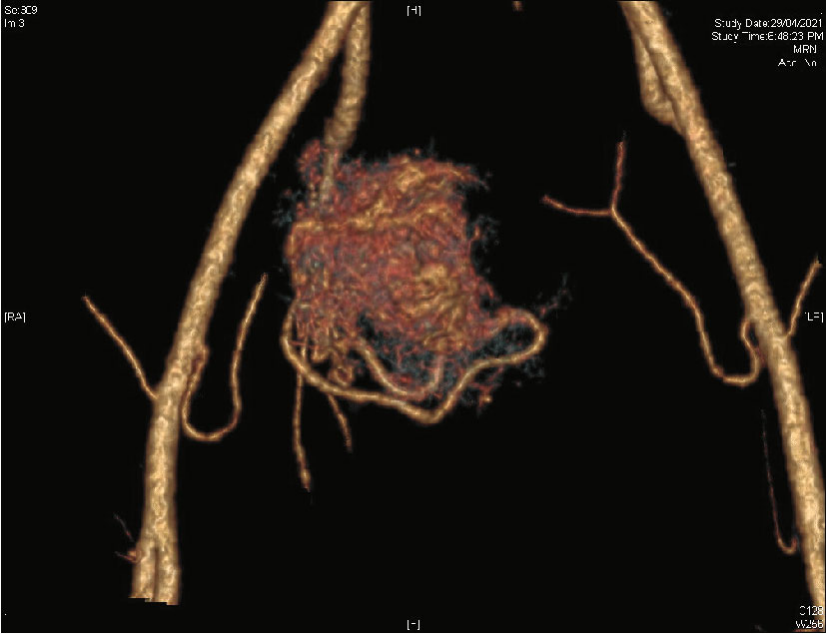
Contrast-enhanced CT angiogram showing the right uterine artery feeding the AVM.
